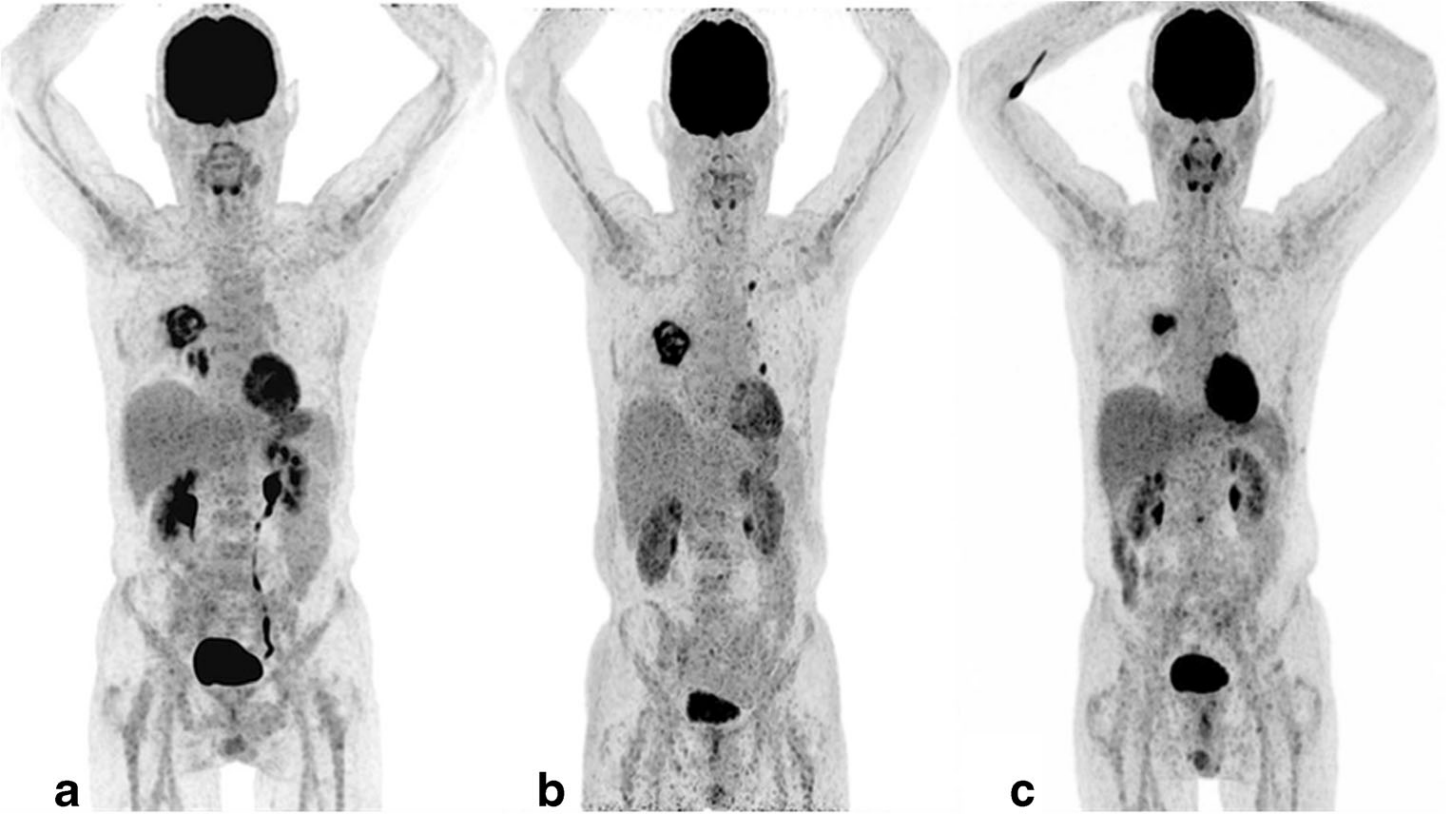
FDG-PET MIP images of a 65-year-old male with AML and diagnosed with aspergillosis from the culture of bronchoalveolar lavage washing (*Aspergillus fumigatus*). Note the complex large heterogeneous pulmonary lesion which did respond but not completely disappear at the final FDG-PET/CT. The patient had a baseline TLG of 144 of the pulmonary lesions. The follow-up scan shows a heterogeneous response, with resolution of the lesions in the right lower lobe below the primary lesion but with appearance of new lesions in the left lung and global TLG of 187. Antifungal therapy was modified, and the last scan showed resolution of lesions except for the primary aspergillus lesion with TLG of 44 after 6 months. This patient had a video-assisted resection of the lesion and subsequently had allogeneic stem cell transplantation (ASCT)
